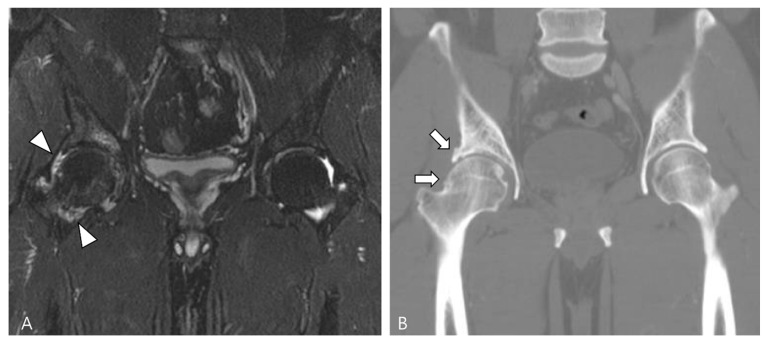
A 47-year-old male with D-TSGCT in the right hip joint. (A) Initial coronal fat-suppressed T2WI shows a tiny low SI nodular synovial thickening in the right hip joint (arrowheads). (B) After synovectomy, follow-up CT after 4 years shows the development of osteoarthritis (arrows) in the right hip joint.
